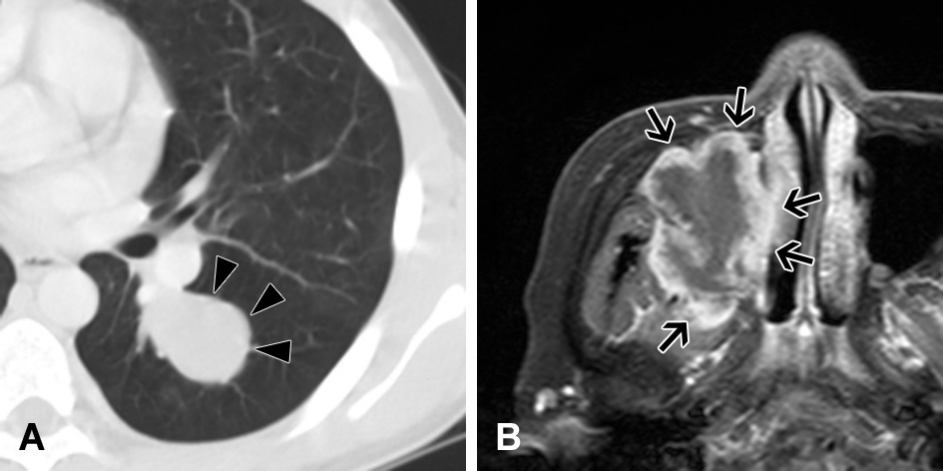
Imaging of inflammatory myofibroblastic tumors A. Chest computed tomography reveals a tumor with smooth and well-defined margins in the lung parenchyma of a 12-year-old boy (Case 2). This tumor is positive for *ALK* rearrangement. B. Head magnetic resonance imaging reveals a lobulated irregular tumor arising in the sinusoidal space of a 70-year-old woman (Case 24). This tumor is negative for *ALK* rearrangement. Resection was incomplete during the first surgery, and the patient experienced local recurrence after 1 month.
